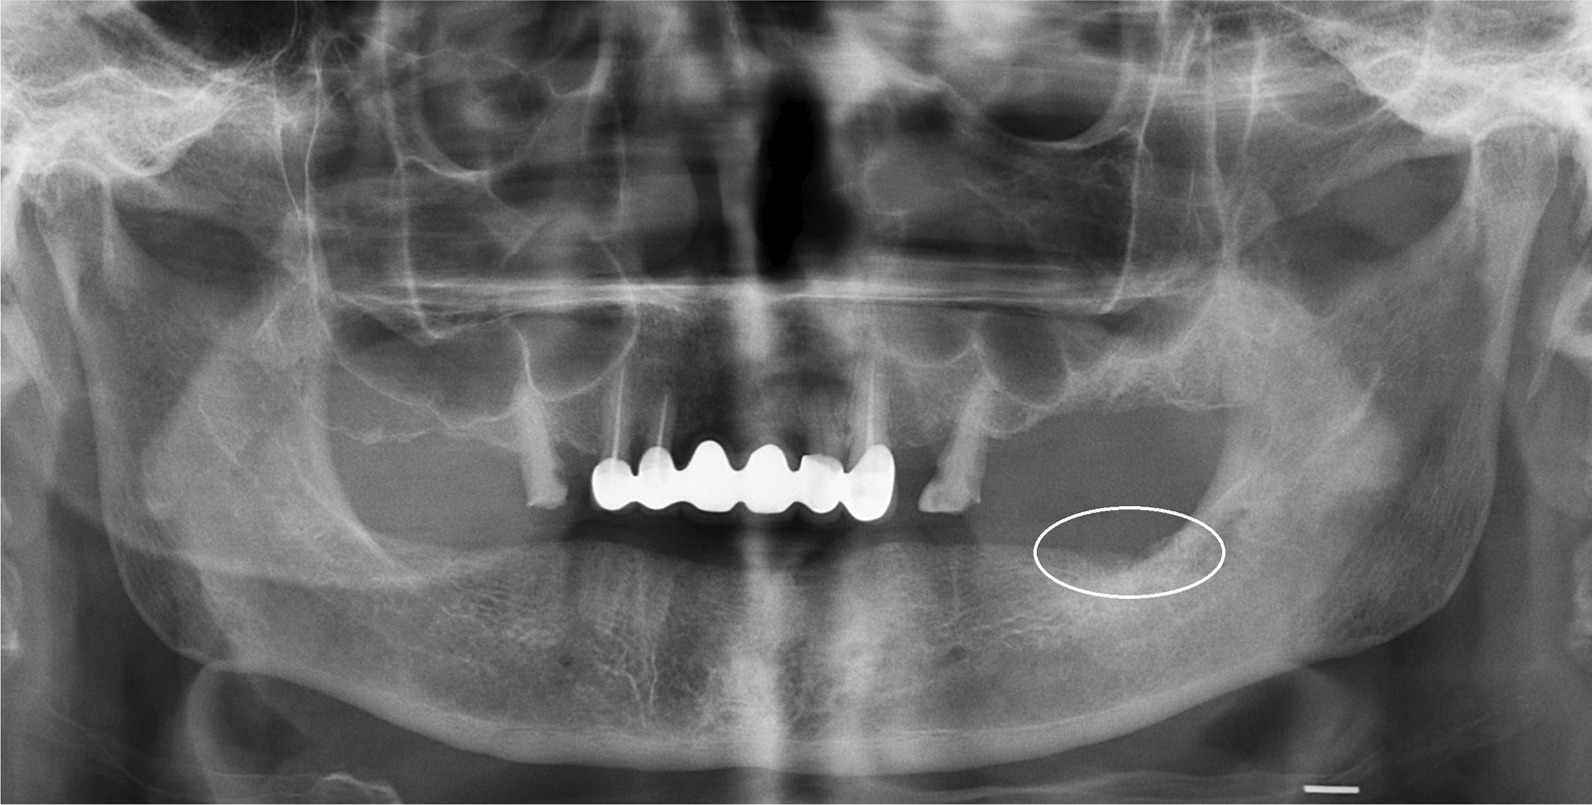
Preoperative panoramic radiograph of a patient with AAOMS Stage II MRONJ lesion on the left, posterior mandible. Encircled area shows the area of osteonecrosis
